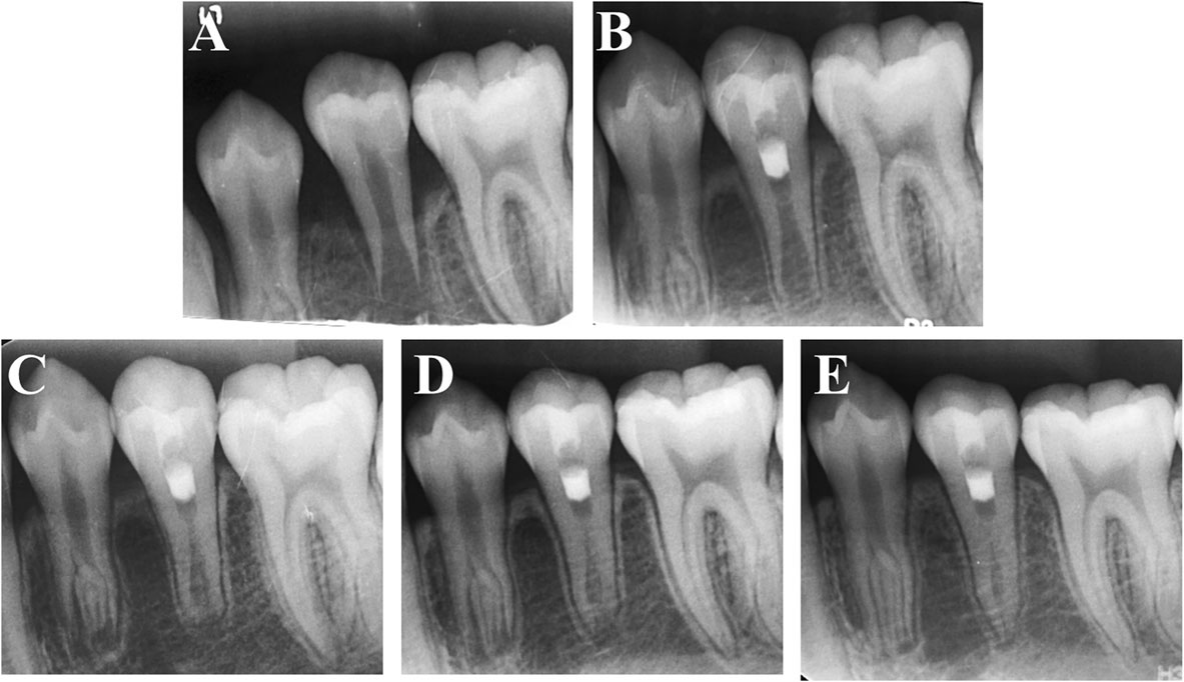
a An intraoral periapical radiograph of #35 with apical periodontitis in a 12-year-old girl. b Three-month follow-up periapical radiograph of tooth #35 after undergoing PRF-aided revascularization. c Six-month follow-up radiograph. d Nine-month follow-up radiograph. e Twelve-month follow-up radiograph showing complete resolution of the periapical radiolucency, thickening of the lateral dentinal walls, and closure of the apex
